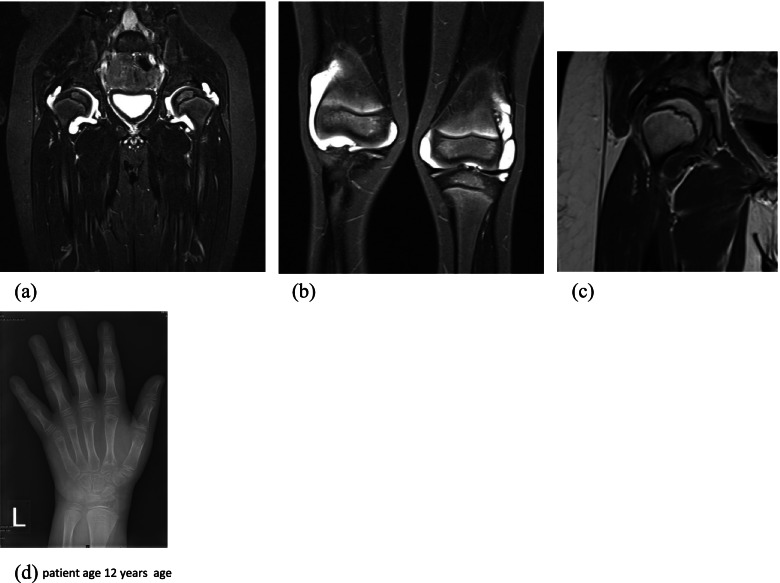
a– b b MRI STIR Coronal T2 sequences show symmetrical effusions affecting both hips a and knees joints, bilateral coxa-vara, with short and broad femoral necks, moderate effusions of the hips and knees (c) Coronal T1 post contrast shows a moderate effusion with minimal synovial enhancement (d) x-ray left wrist showing periarticular osteopenia
